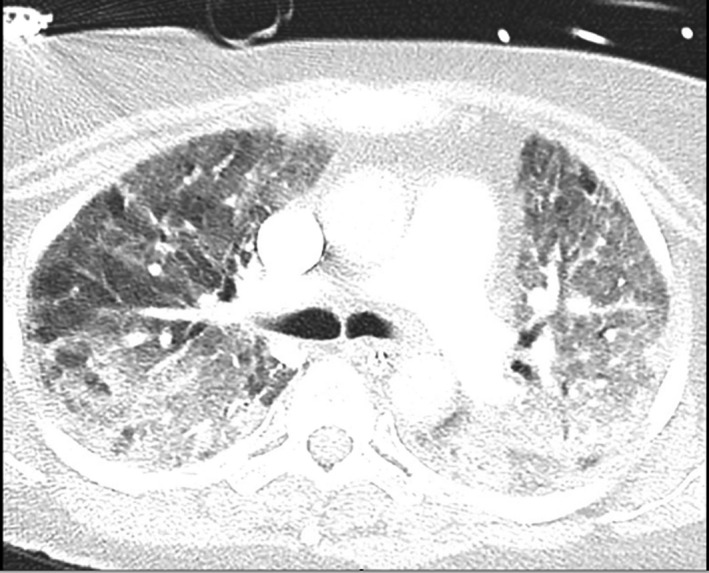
CT chest demonstrating extensive consolidation and ground glass opacification
